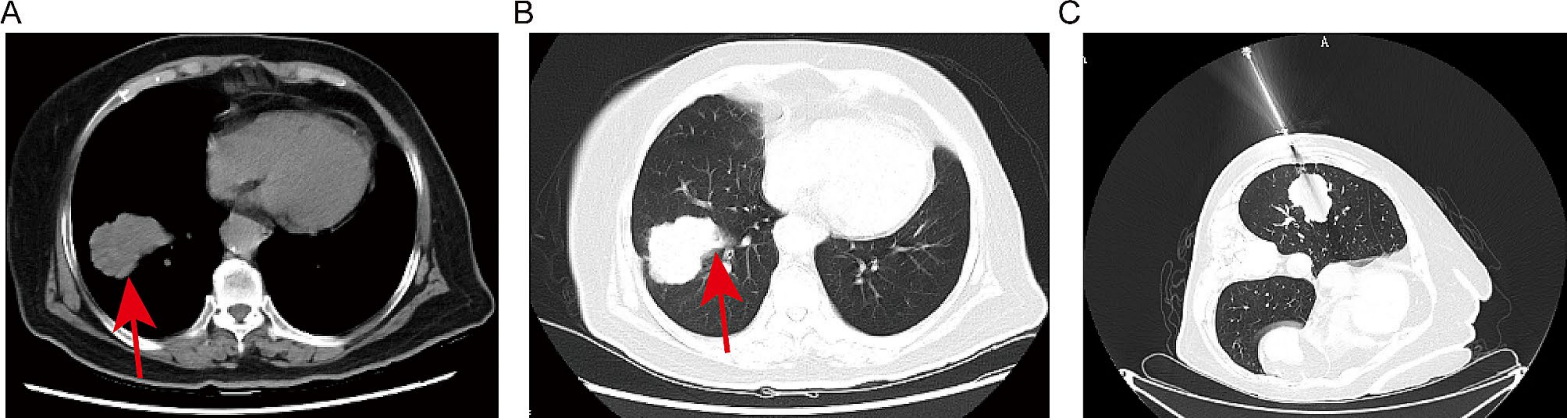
CT scan showed a tumor mass of irregular border in the lower lobe of the right lung. (A) Mediastinal window and (B) lung window showed a right-side lung mass (arrow indicated the location of lesion); (C) The localization needle inserted into the chest wall indicated the needle entry route
